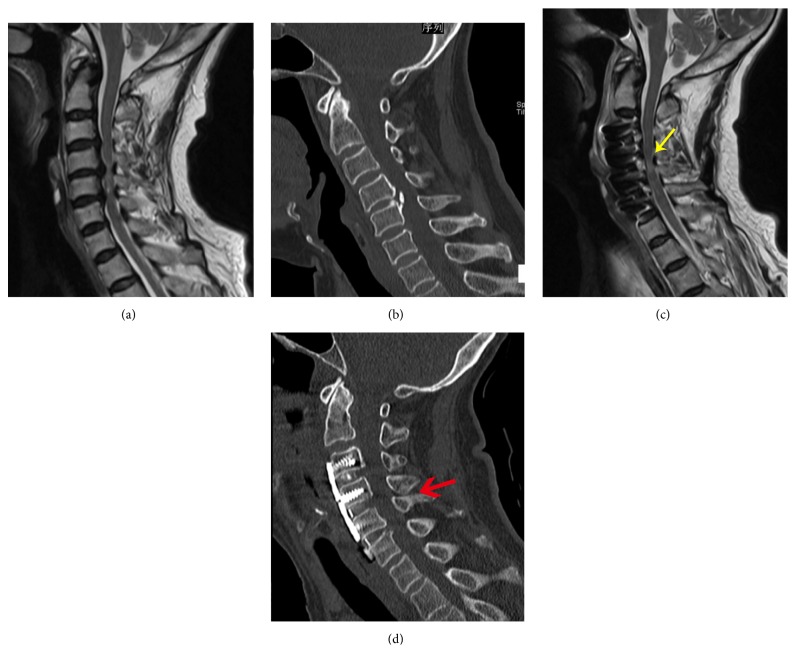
Female, 70-year-old, weakness of limbs for 1 year. Preoperation JOA score was 10. Preoperation Cobb's angle was 10.57° in lateral cervical X-ray. The MR scanning showed C3/4, C4/5, 5/6-disc herniation with posterior wrinkled and incrassated ligament Flava, leading to pincer spinal stenosis. The anterior and posterior occupying rate were 45.4% and 33.3% (a). The sagittal CT imaging showed ossification of the posterior longitudinal ligament (OPLL) in C4/5. (b). The spinal cord was decompressed sufficiently by ACDF of C3/4, C4/5, 5/6 (c). The OPLL was excised by ACDF without corpectomy, while the cage of C4/5 was implanted closer to the anterior vertebral body, leading to intervertebral plate height of C4/5 loss. The wrinkled and incrassated ligament Flava of C4/5 still existed (c&d). Cobb's angle was 28.78°. Postoperation JOA score was 16 at 6th-month follow-up.
